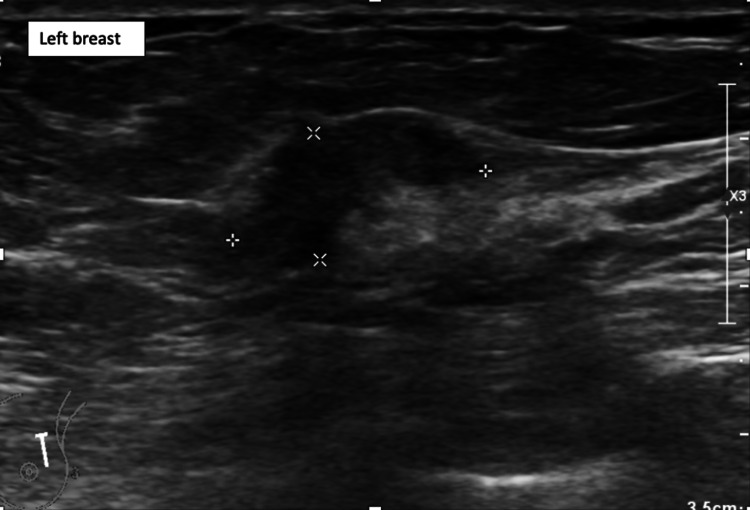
Ultrasonography of the left breast showing U5 lesion
